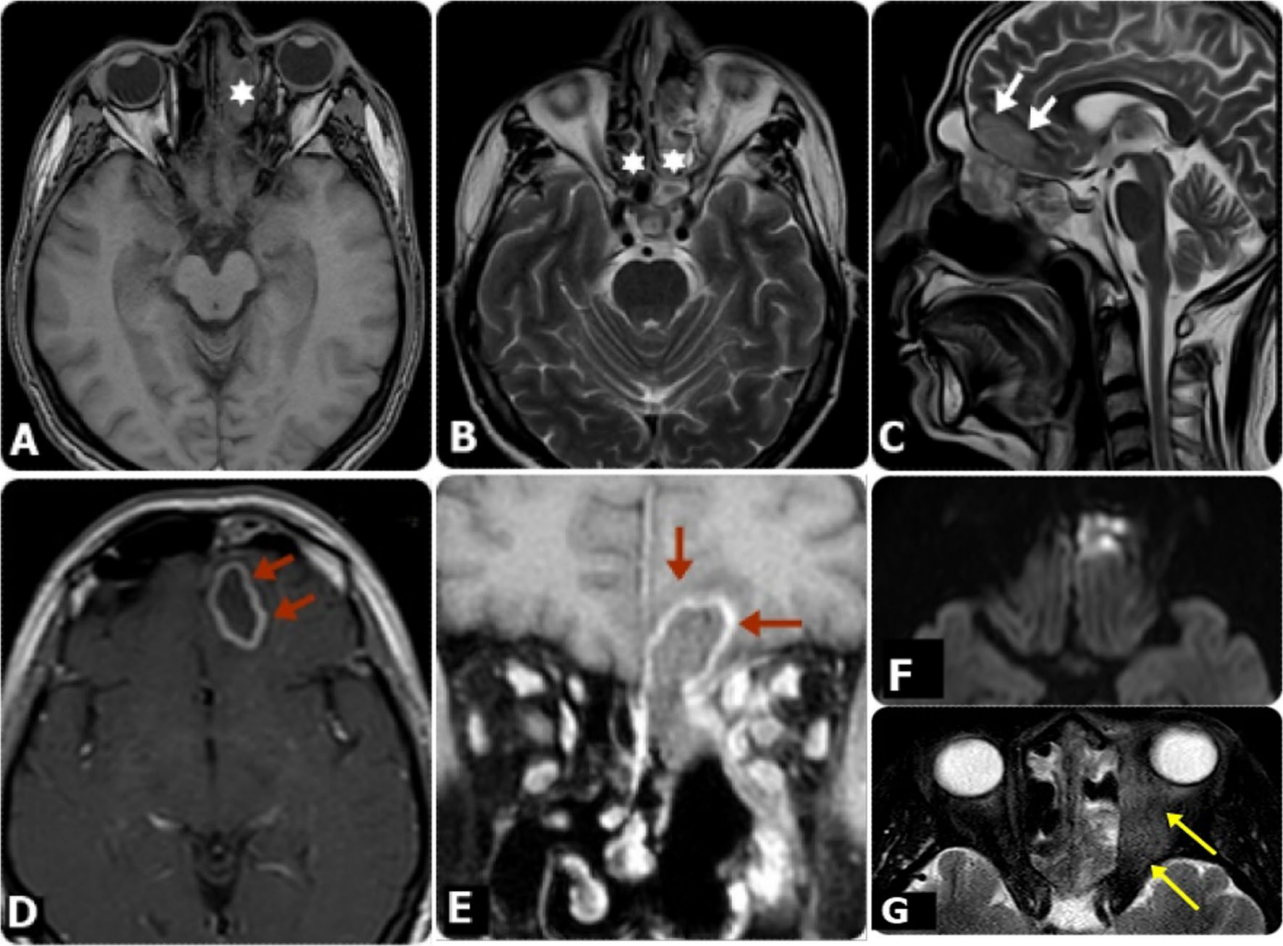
MRI findings in diabetic male patient, 59 years old, presented mainly with headache and altered mentation, in addition to Lt. eye proptosis, with periocular and eyelids edema, following COVID-19. A Axial T1W, B and Axial T2W images showed bilateral ethmoidal sinusitis (Asterisks), more evident at the Lt. side, with extension of the inflammatory process to the Lt. orbit. C Sagittal T2 WI, showed extension of the inflammatory process through the cribriform plate to the Lt. basi-frontal lobe (White arrows), exhibiting an area of high signal intensities, as well as frontal sinusitis. D Axial post-contrast T1WI and E coronal post-contrast fat-saturated T1WI showed marginally enhanced lesion at the Lt. basi-frontal lobe, which presented restricted diffusion on DW images at b-value 1000 (F), in keeping with cerebral abscess (Red arrows). Coronal post-contrast fat-saturated T1WI showed also extension of the inflammatory process to the Lt. orbit, resulting in mild enlargement of the related Lt. extra-ocular muscles. G Axial T2 SPIR image shows Lt. retro-ocular fat stranding (Yellow arrows)
